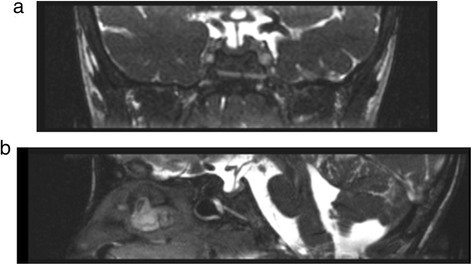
Magnetic resonance imaging of the hypothalamic-pituitary region. Flattened pituitary gland. a coronal view. b sagittal view
